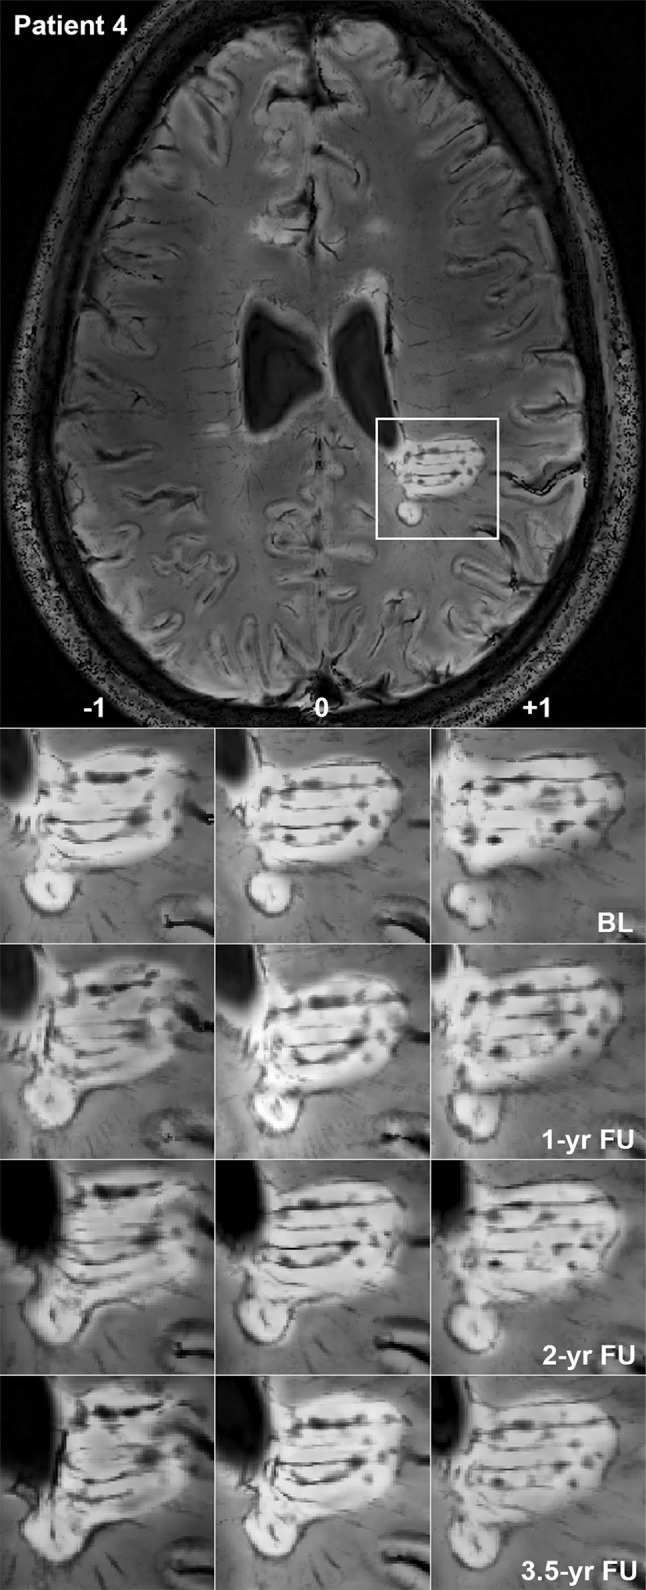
Expansion of a rim lesion. Patient 4, a 29-year-old lady with relapsing-remitting multiple sclerosis lasting for 5 years, EDSS 2. 7 T FLAIR–SWI data show several WM hyperintense lesions typical for the disease. One large periventricular hyperintense lesion with an encircling hypointense rim is indicated by a *white rectangle* and magnified. Within this lesion, tubular hypointense structures suggestive of veins and circumscribed nodular hypointensities are visible. Images demonstrate a slow expansion of the posterior parts of this lesion over 3.5 years, leading to a fusion of initially separated lesion parts. Three contiguous imaging slices (−1, 0, +1) show that expansion and fusion are not due to willful slice sampling. Note the global brain atrophy of this patient over time, which is evident from the widening of the ventricles. *BL* baseline, *FU* follow-up, *yr* year
